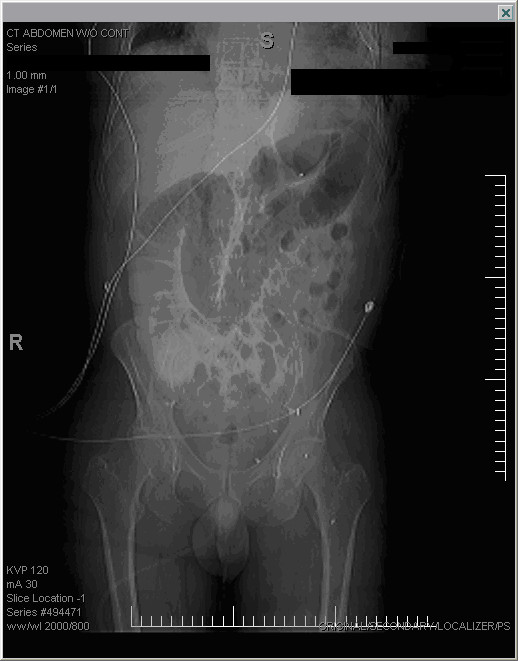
Upright CT abdomen without contrast obtained 24 hours after colonoscopy. Upright view shows herniation of large bowel through left hemidiaphragm and into thoracic cavity.
